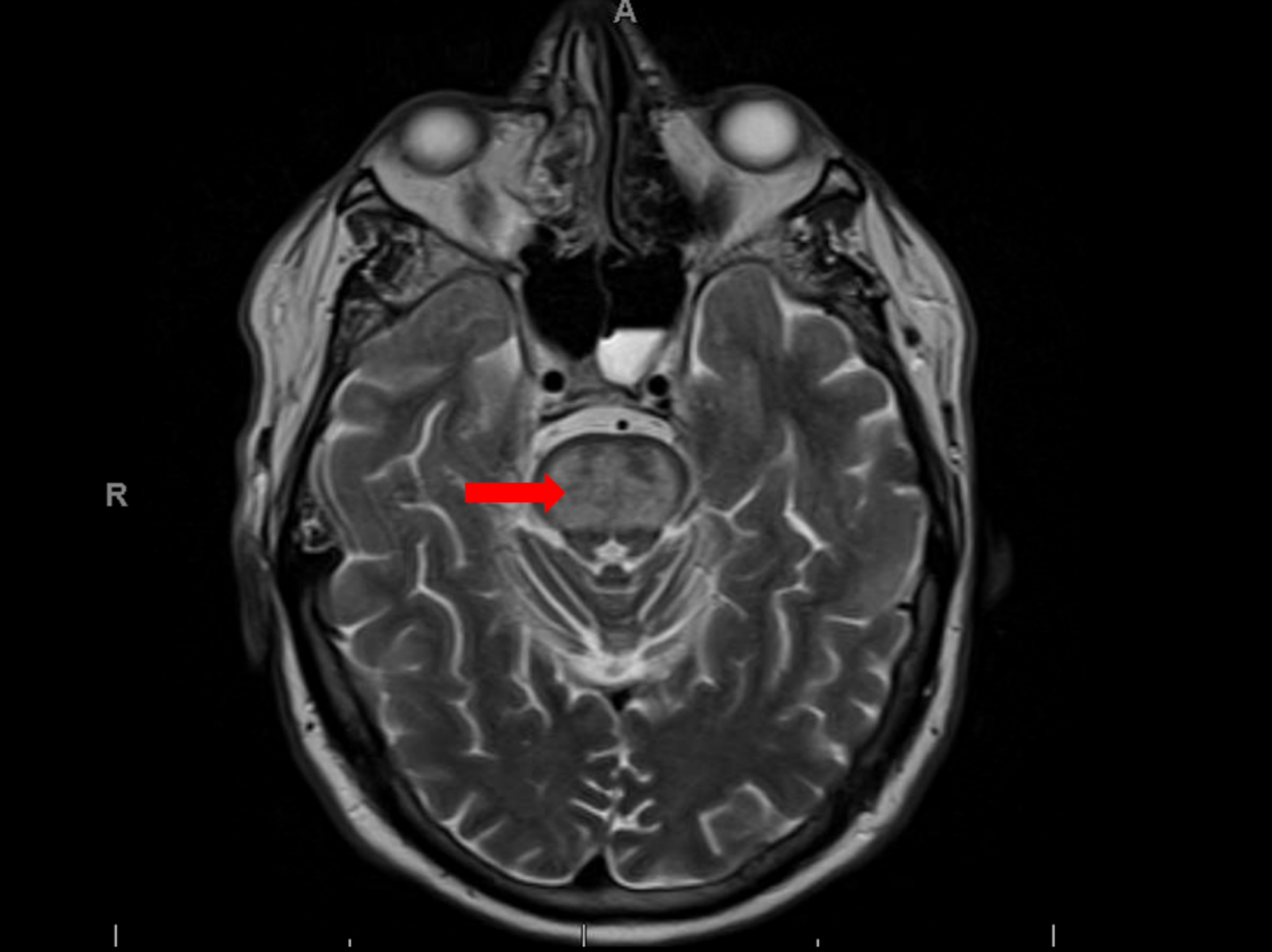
Magnetic resonance imaging revealed edema and swelling in the pons (red arrow) consistent with pontine demyelinosis
